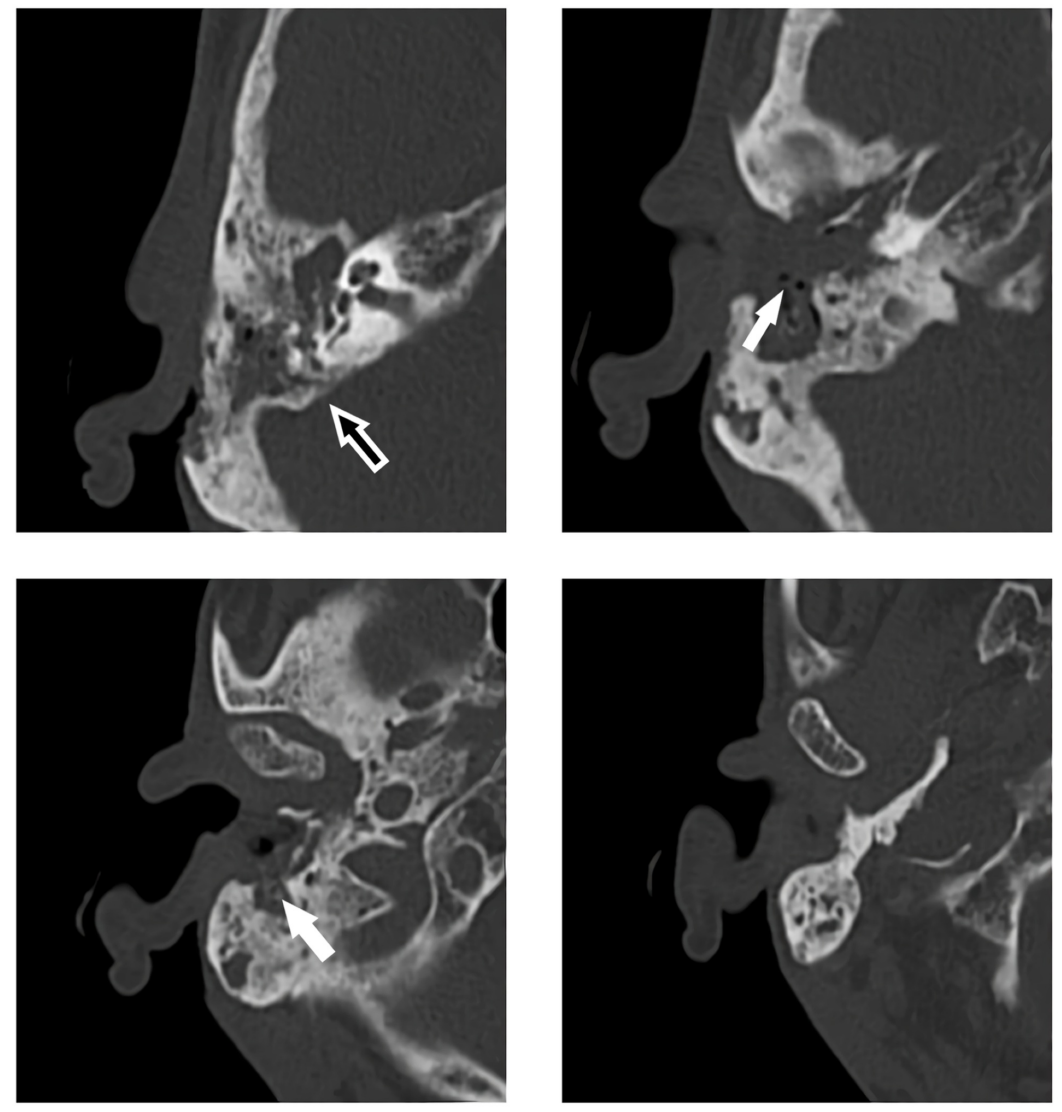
CT scan (axial plane) of mastoid with erosive infestation of the anterior (white arrow) and posterior (black arrow) bony wall of the external ear canal, focal dehiscence of the cranial wall of the mastoid, and opacification of the mastoid air cells and middle ear.
